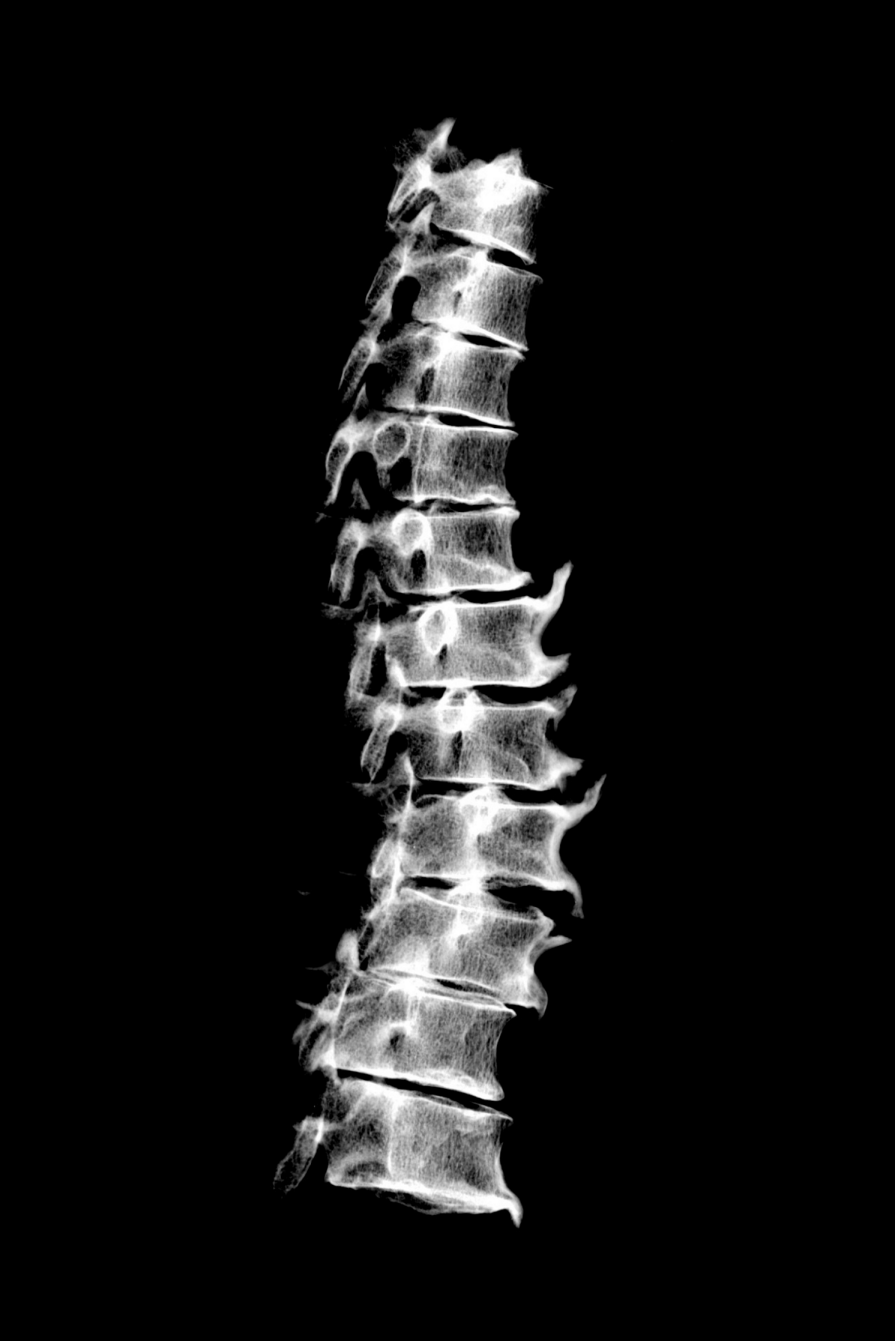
Radiograph of the T2–T12 thoracic vertebrae (*norma lateralis*). Note the ossification at the site of the anterior longitudinal ligament that coats the vertebral bodies.
